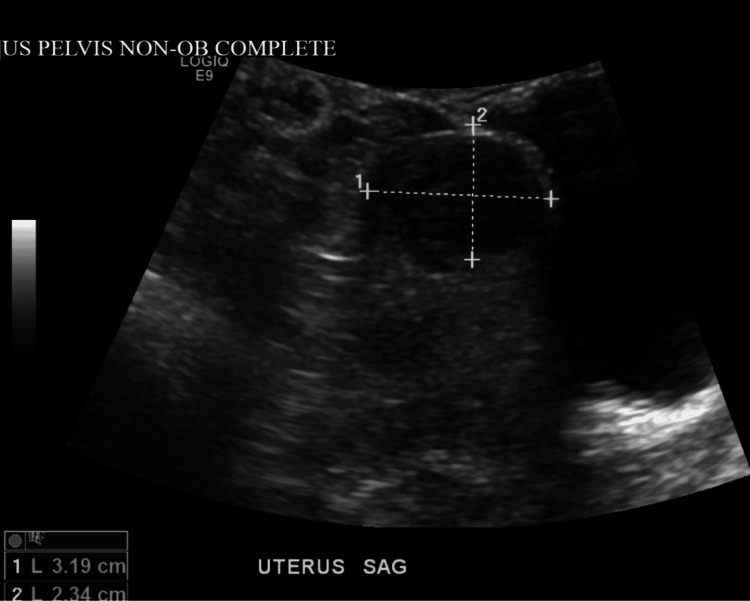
Pelvic ultrasound showing a 3.2 centimeter intramural fibroid at the uterine fundus
